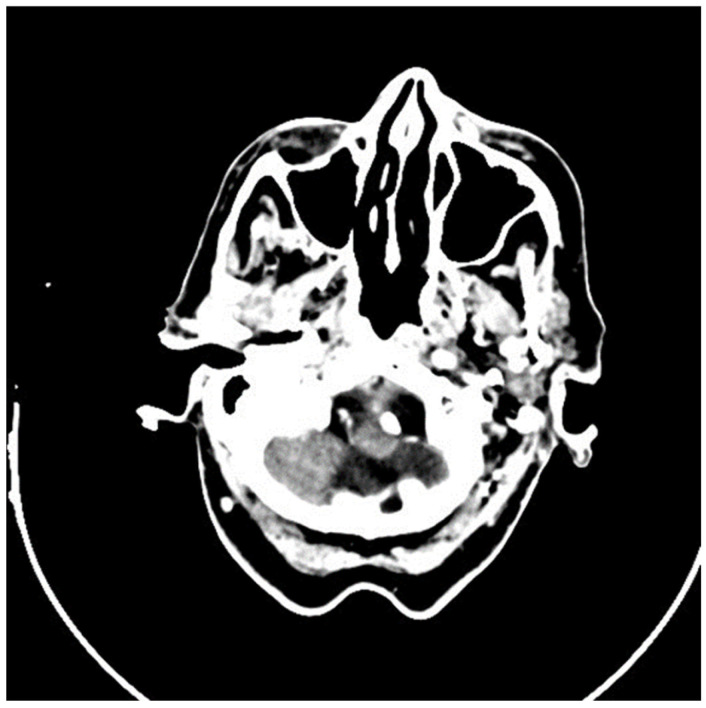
Preoperative CT imaging showing tumor involvement in the left nasal region.
